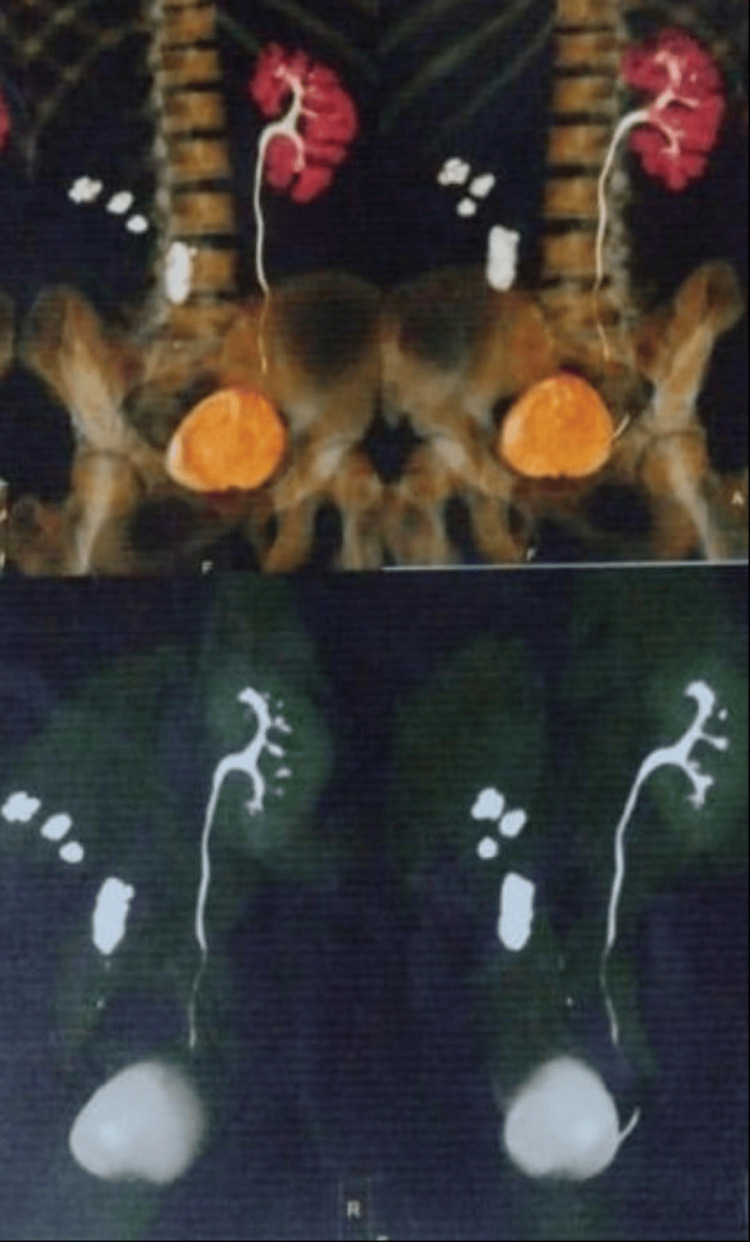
CT pyelography showing non-functioning right kidney with renal and ureteric calculi
